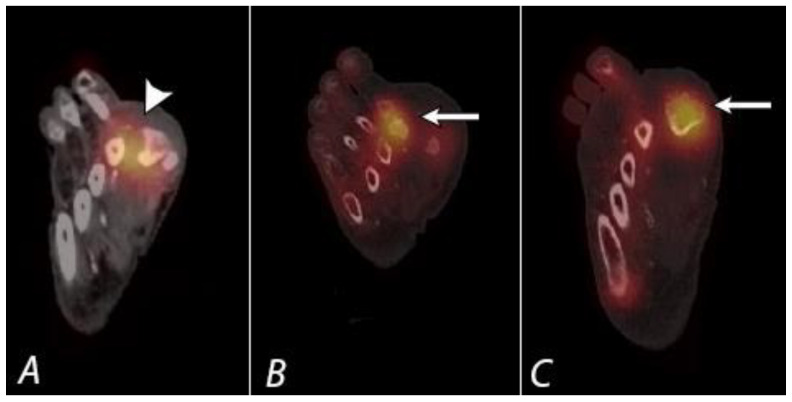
Fifty-eight-year-old diabetic male with history of right great toe amputation (at the MTP joint) 2 months previously, now with new pain, swelling, and redness in the medial forefoot. ^99m^Tc MDP SPECT/CT bone scan (B,C) shows increased uptake at the first metatarsal head and the second MTP joint (white arrows). An ^111^In WBC SPECT/CT scan (A) shows concordant uptake as well as a larger area of soft tissue uptake around first metatarsal head and the second MTP joint, confirming cellulitis in addition to osteomyelitis (white arrowhead).
